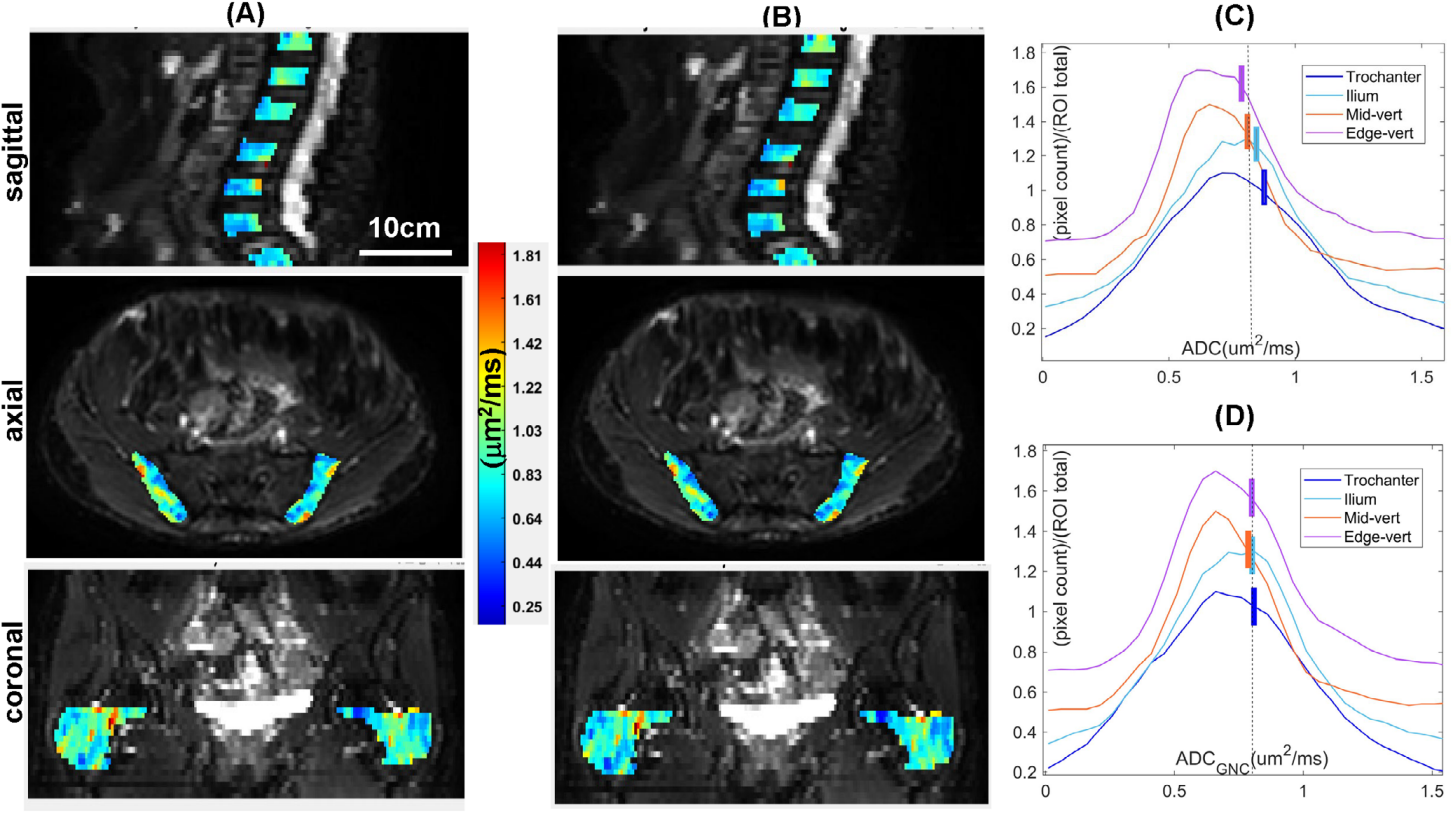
The ADC map ROI overlays on *b* = 0 s/mm^2^ DWI are shown for a representative myelofibrosis study subject in three orthogonal planes (arranged vertically) before (A) and after (B) gradient nonlinearity correction (GNC). The ADC maps were smoothed with 3‐point median filter. Color‐bar indicates the common ADC scale. The corresponding ADC histograms (bin size 0.05 μm^2^/ms, normalized to total count) are shown before (C) and after GNC (D) as stack plots (vertically offset for clarity) across trochanter, ilium, mid‐vertebrae (L2‐L4), and edge‐vertebrae (T12, L1, L5, and S1) bone sites (color‐coded in the legends). The colored notches mark the mean ADC values with respect to nominally bias‐free ADC(L3)‐reference (dashed vertical line).
